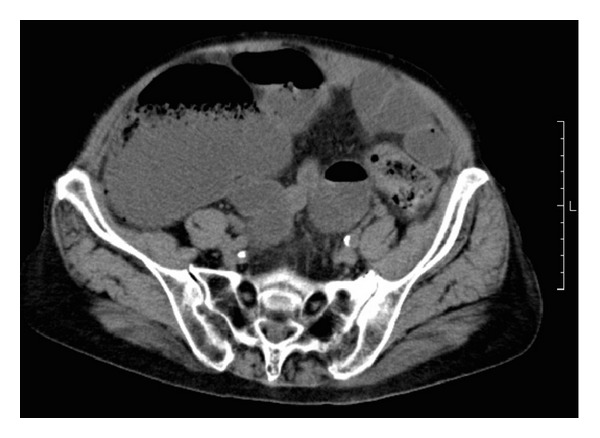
Plain computed tomography scan shows a dilated cecum measuring 95 mm.
